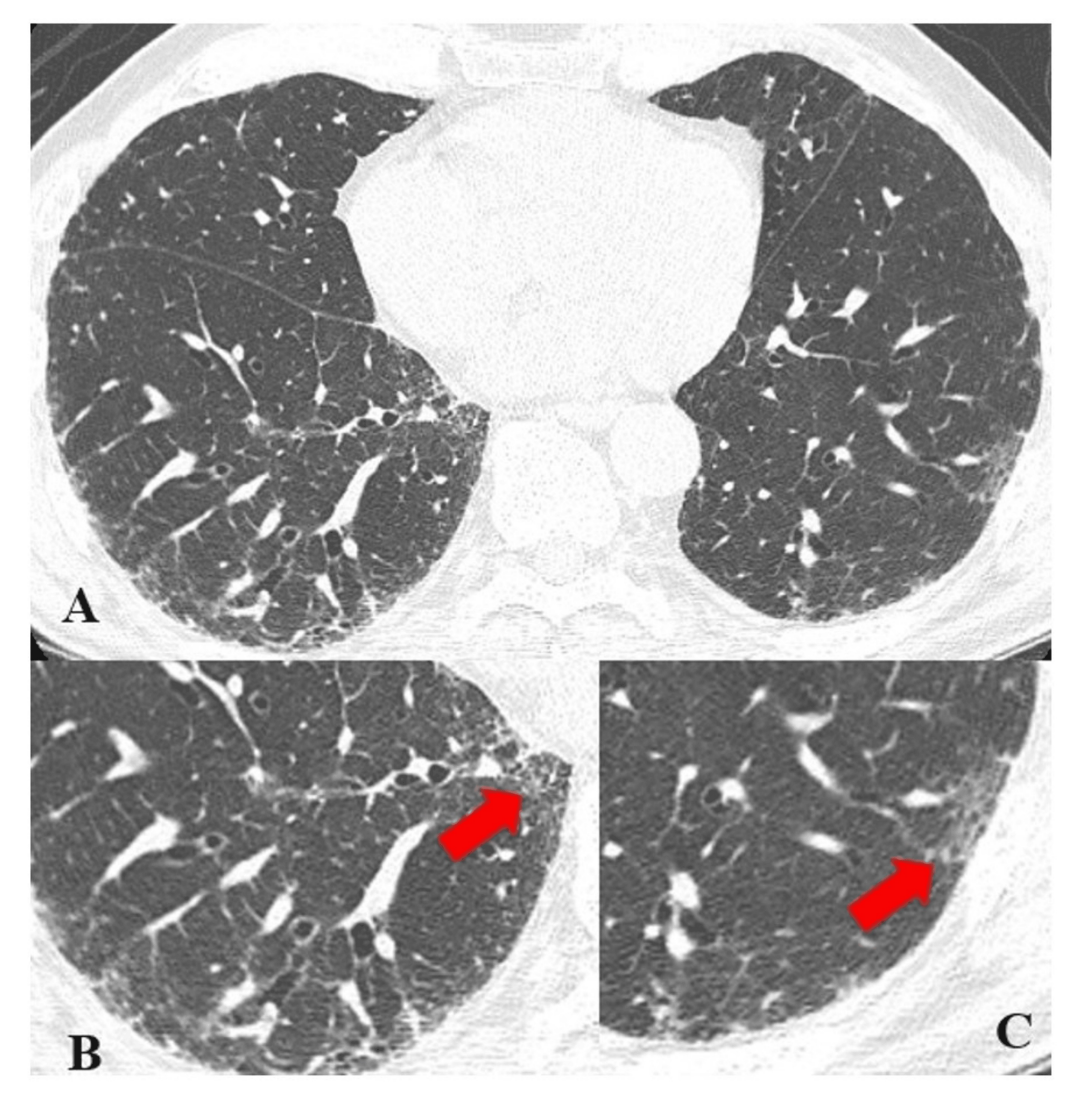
Mild ILD reticular opacities with peripheral/subpleural distribution (A) Axial CT image of the chest and magnified images of the periphery of the lung bases. (B and C) Small areas of reticular opacity (arrows) in this case, with bilateral subpleural distribution. ILD: interstitial lung disease, CT: computed tomography Image credit: Dr. Jordan Ditchek, MD (used with permission)
